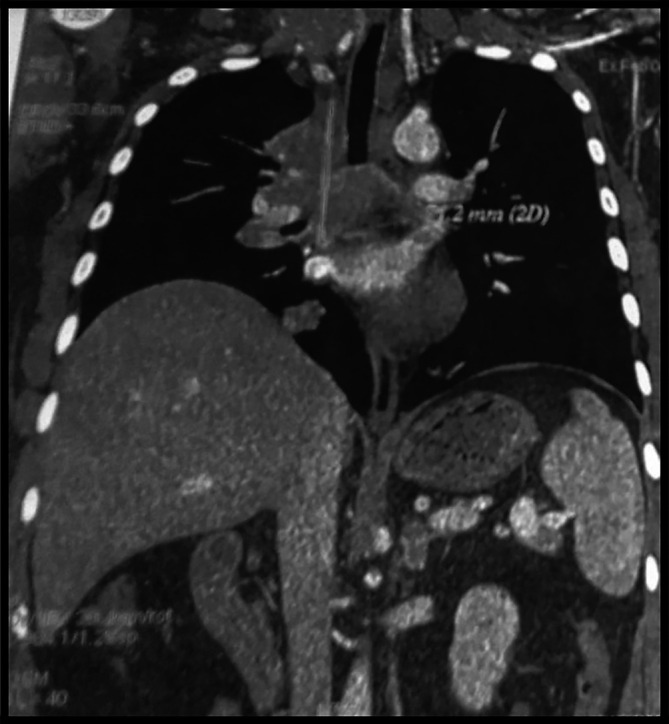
Coronal section of the computed tomography chest demonstrating a bulky tumor invading the carina, the right main bronchus, the pericardium with a subpleural right node.
